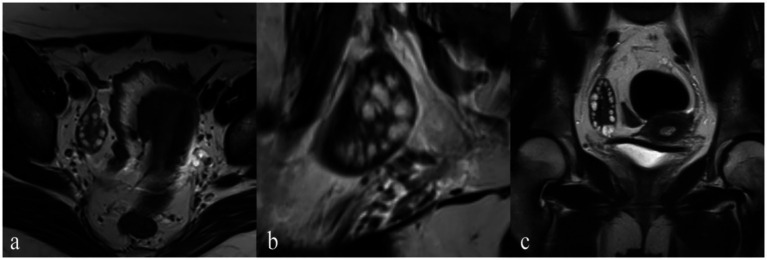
A PCOS patient with amenorrhea, acne, and hirsutism (Ferriman-Gallwey Score: 12). The patient has 36 follicles, and the ovarian volume is 22 cm^3^. (a) Axial T2-weighted imaging (T2WI) provides a cross-sectional view emphasizing the distribution of the follicles. (b) Sagittal T2WI displays the ovaries with multiple follicles. (c) Coronal T2WI displays the view highlighting the follicles’ arrangement and size.
